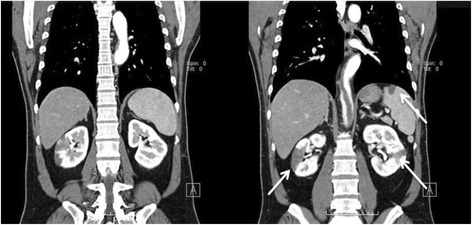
Preoperative contrast-enhanced computed tomography. The computed tomography scan showed both the multiple renal and splenic infarctions (*white arrows*) and the large intra-aortic thrombus in the descending thoracic aorta
